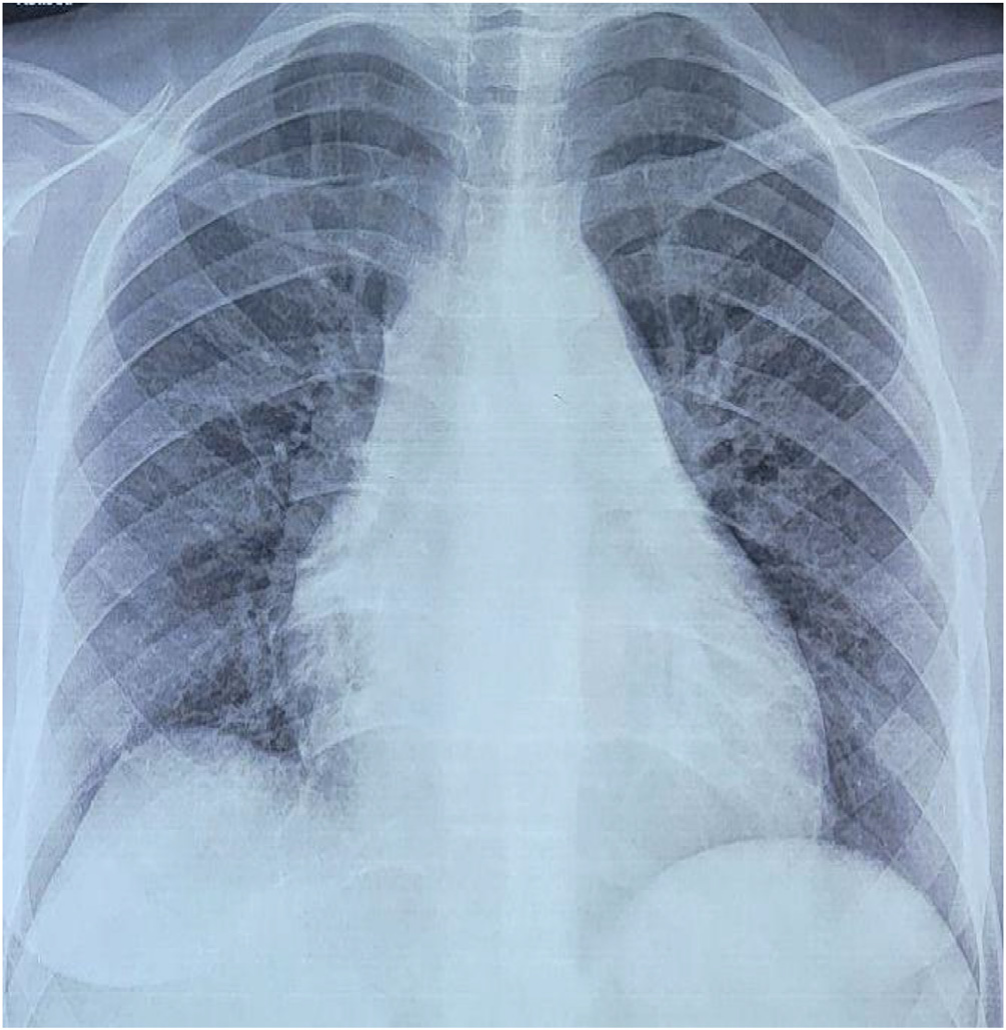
Frontal chest X-ray showed moderate cardiomegaly, leftward convexity of the mid-heart shadow.
